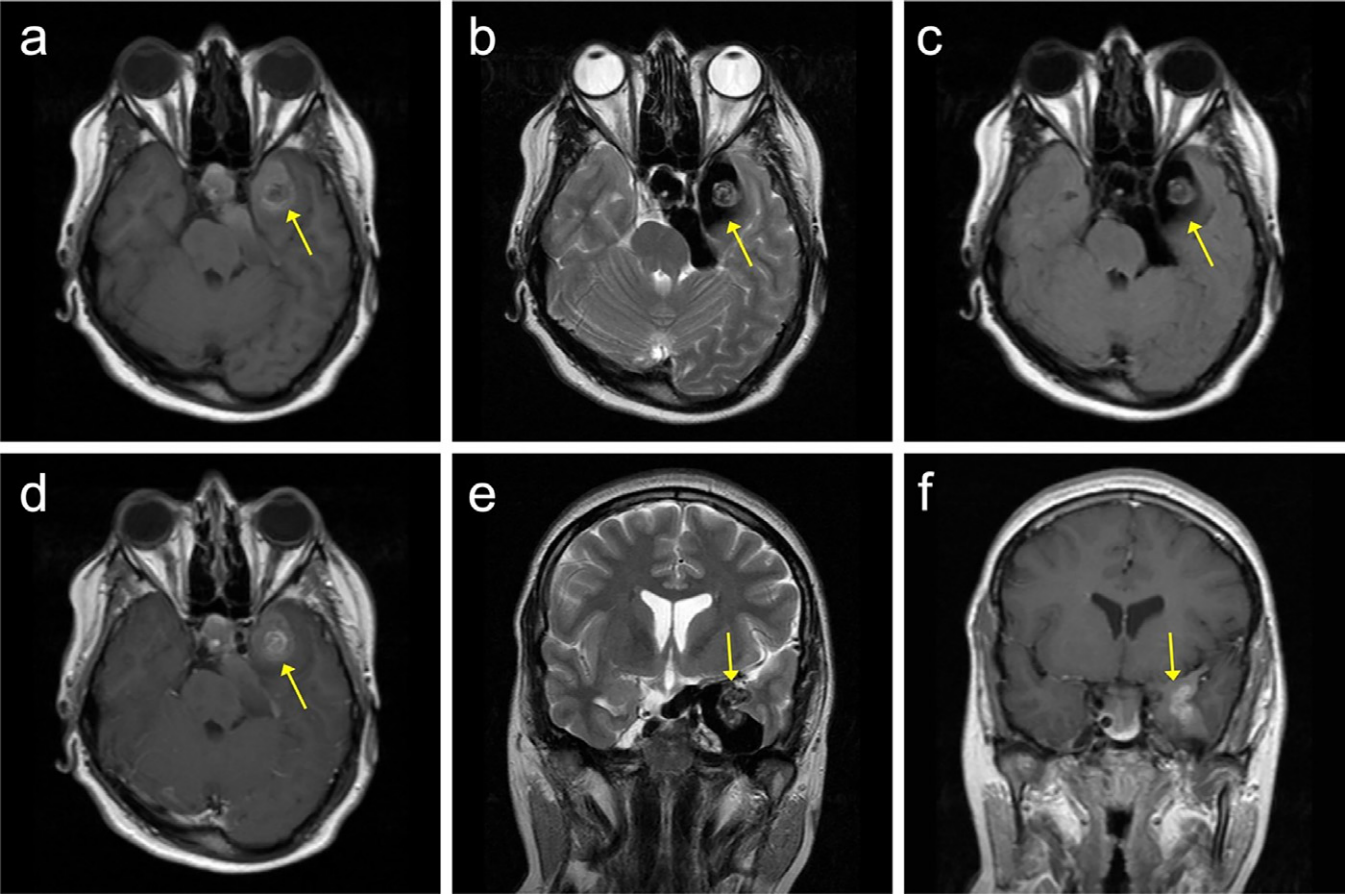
Brain MRI with and without GAD. (A) Axial T1W (B) Axial T2W (C) Axial T2 FLAIR (D) Axial T1W with GAD (E) Coronal T2W (F) Coronal T1W with GAD. Evidence of a lobulated nonenhancing heterogeneous mass with High signal intensity on T1 and low signal intensity on T2 and FLAIR images is present in the left middle cranial fossa, with pressure effects on the left temporal lobe and left side of the pons. The epidermoid cyst is indicated with a yellow arrow in all sequences.
